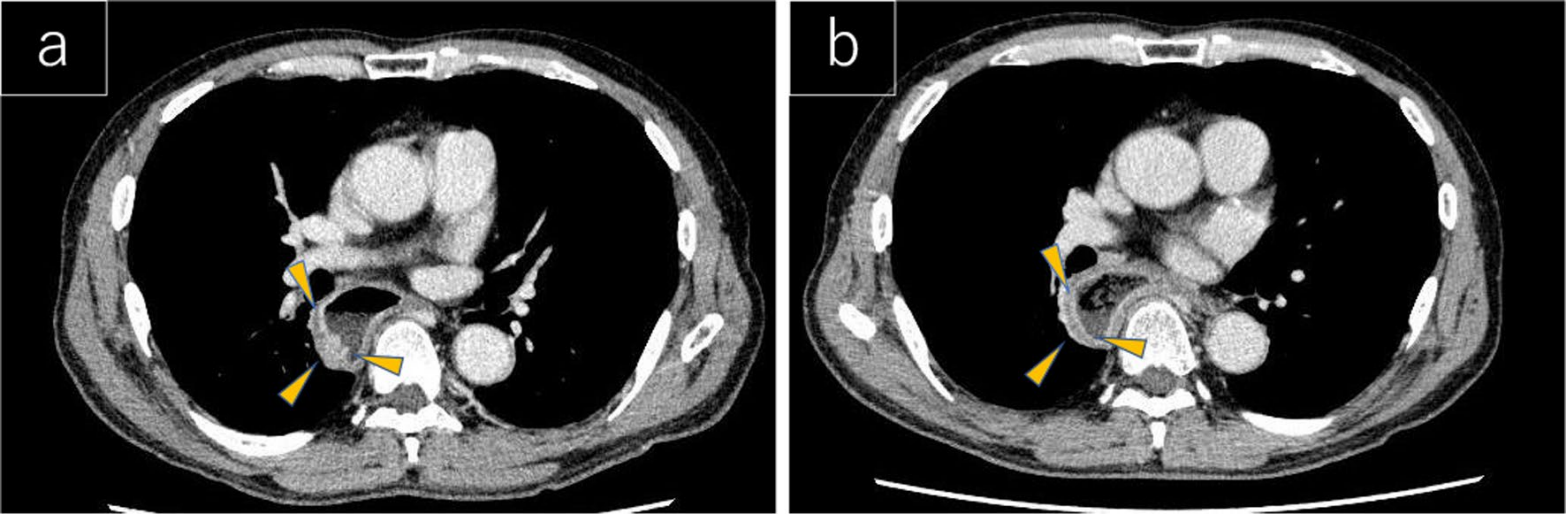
Computed tomography images showing middle thoracic esophageal wall thickness (arrow head), a before neoadjuvant chemotherapy and b immediately before surgery
